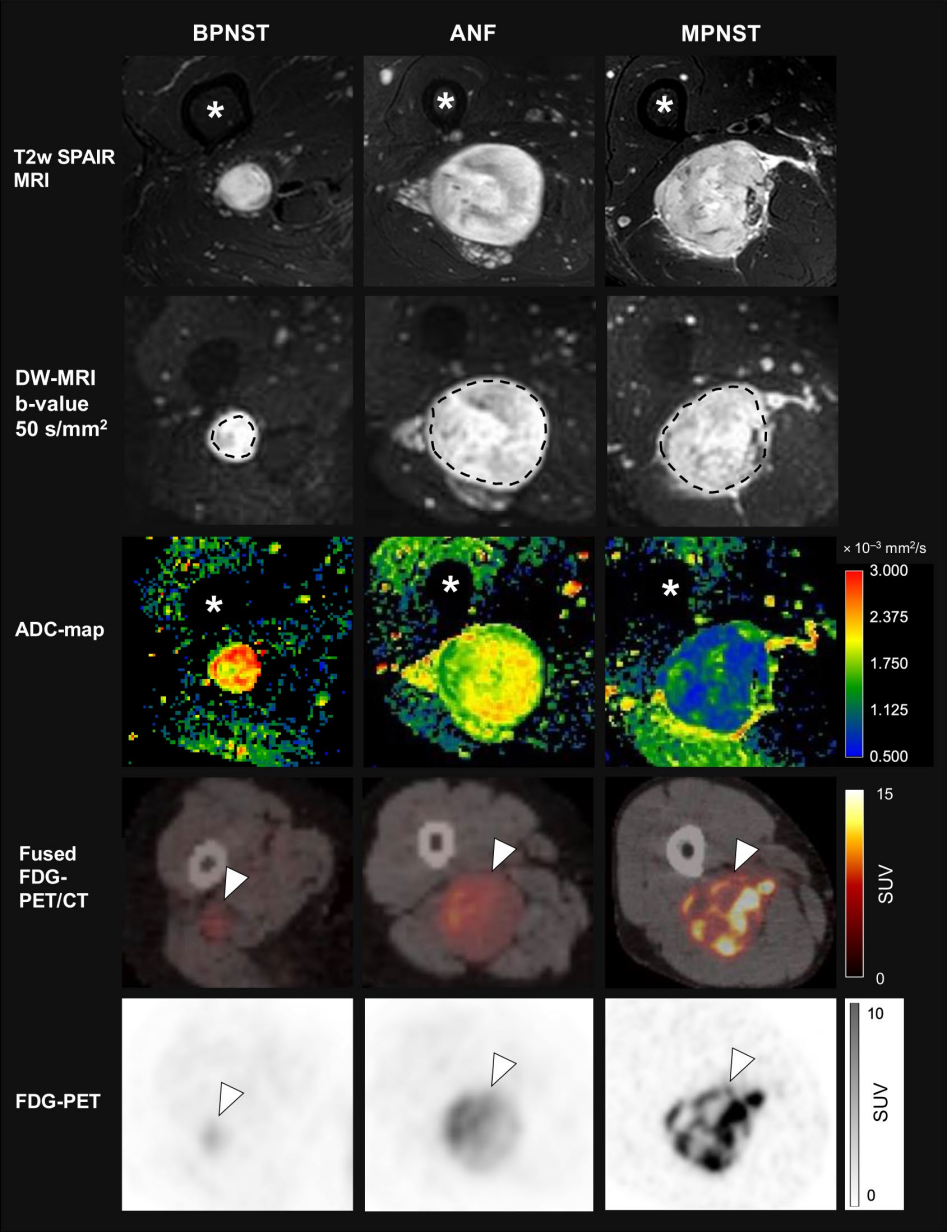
Comparison of T2-weighted SPAIR MRI, parametric ADC maps, and F-18-FDG-PET/CT in three different NF1 patients with nerve sheath tumours of the thigh. Left column: BPNST (arrowhead) in a 54-year-old female; middle column: ANF (arrowhead) in a 49-year-old female, right column: MPNST (arrowhead) in a 31-year-old male. First row: transverse slices of a T2-weighted SPAIR sequence at 3T (* depict the femoral bone); second row: ROI contouring along the tumour margins in diffusion images (b-value 50 s/mm^2^); third row: corresponding parametric ADC maps; fourth row: fused F-18-FDG-PET/CT; fifth row: F-18-FDG-PET. BPNST = benign peripheral nerve sheath tumour; ANF = atypical neurofibroma; MPNST = malignant peripheral nerve sheath tumour; SUV = standardized uptake value
